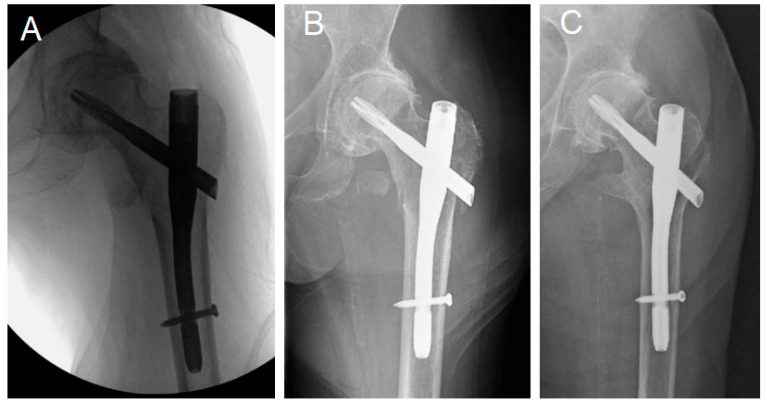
(A,B) Intraoperative and Immediate post. (C) 71-week postoperative radiographs of a 91.8-year-old female with a wide medullary canal and uneventful fracture healing without nail toggling despite a proximal lateral wall fracture.
